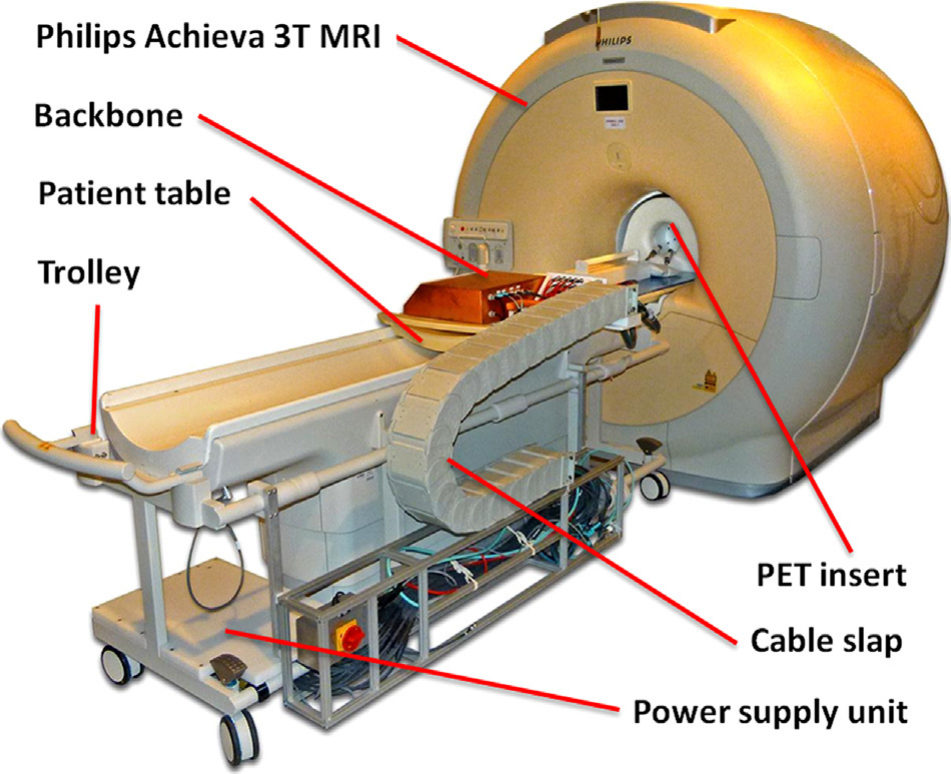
Hyperion-II^D^ PET insert with 10 PET modules (2 stacks each) mounted on the patient table top of a 3T clinical MRI.
